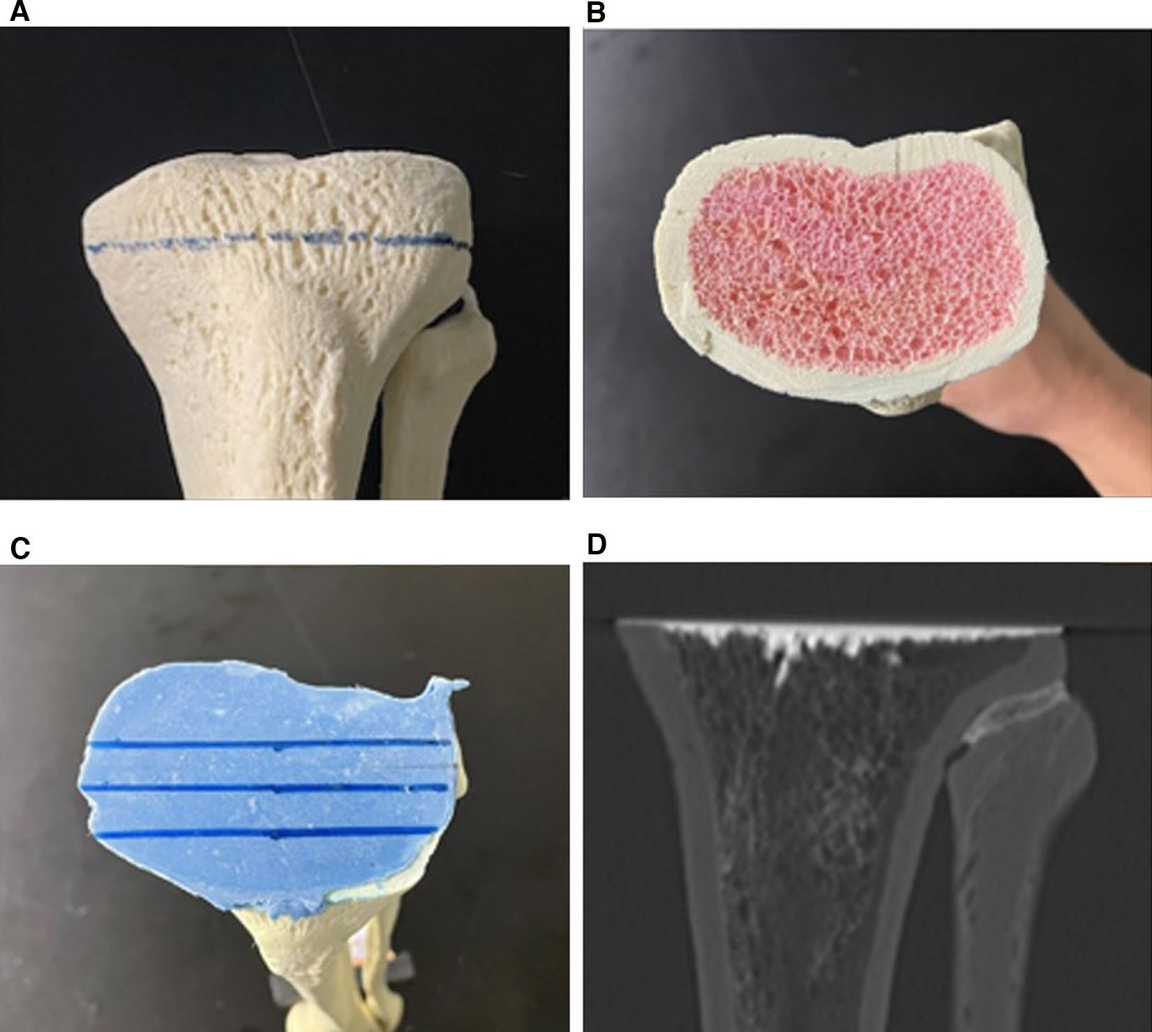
Preliminary study to evaluate cement penetration using a bone model. A Bone model of tibia. The osteotomy line was set as observed in total knee arthroplasty. B Osteotomy surface of the bone model. C Bone model after cement application. Cement penetration was measured at each of the cross-sections where the lines were drawn. D Computed tomographic assessment on the bone model. The same method as in Fig. 1 was used to evaluate the mean and maximum cement penetration
